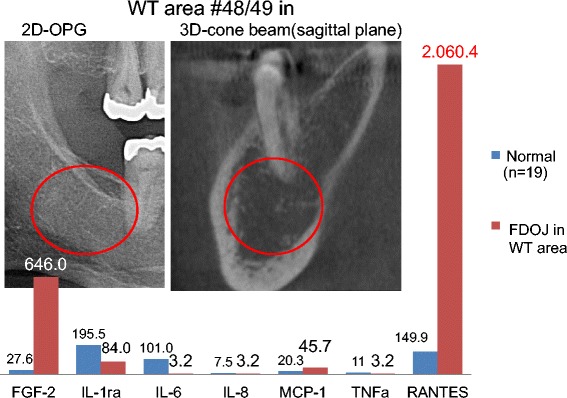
Comparison of seven cytokines in the FDOJ in area #48/49 with cytokines in a healthy jawbone (*n* = 19). The left X-ray shows the retromolar WT area on a two-dimensional orthopantomogram (2D-OPG); the right X-ray shows the same area in the sagittal plane in three-dimensional cone beam (3D-CB).
